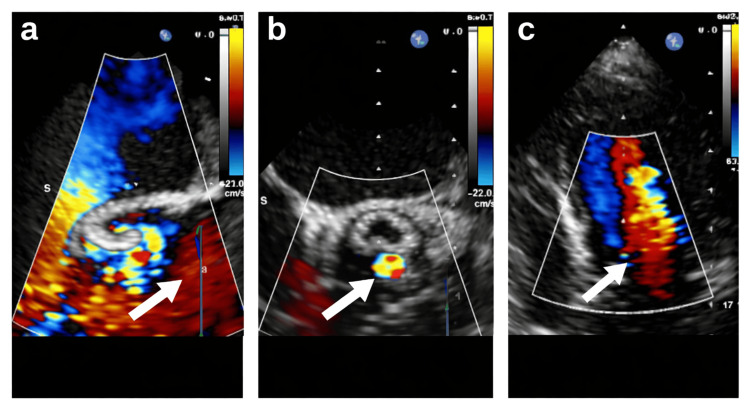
Echocardiographic evaluation of prosthetic valve dysfunction and post-procedural outcome. (a) Pre-procedural transesophageal echocardiography demonstrating severe transvalvular aortic regurgitation, with a prominent color Doppler jet directed into the left ventricle (arrow). (b) Transesophageal short-axis view demonstrating paravalvular leakage around the prosthetic valve visualized by color Doppler imaging (arrow). (c) Post-procedural transthoracic echocardiography demonstrating resolution of severe regurgitation after implantation of the third valve, with only mild residual paravalvular leakage (arrow).
